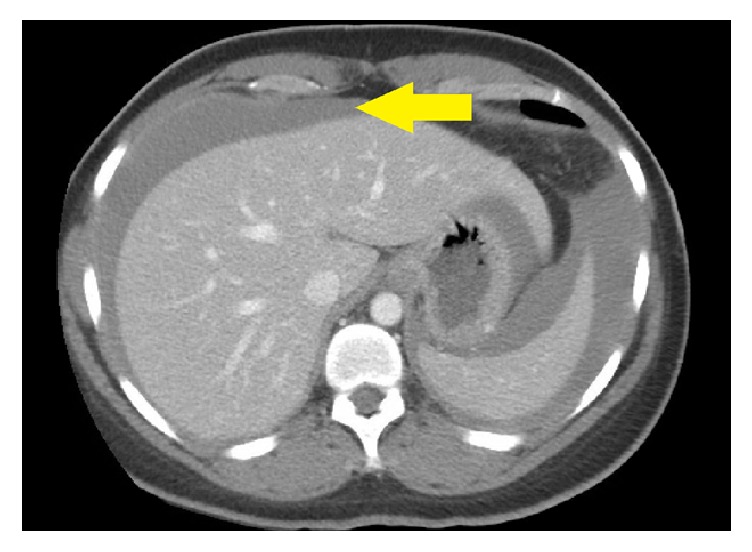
CT of the abdomen with blood surrounding the liver and spleen (arrow).
